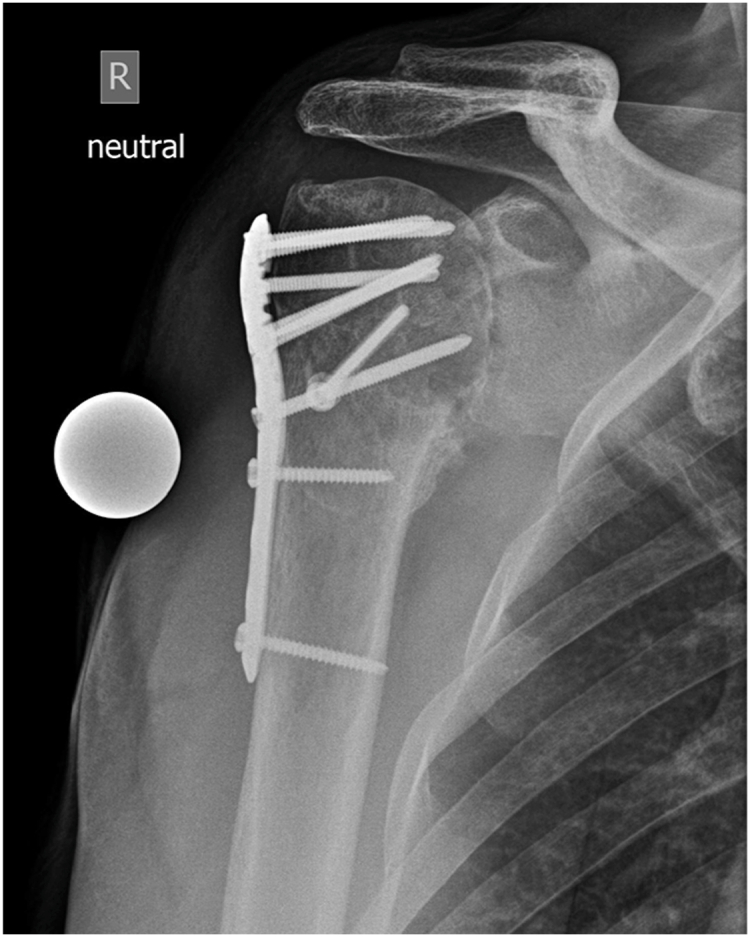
Anteroposterior radiograph of the right shoulder before revision surgery showing glenohumeral osteoarthritis and humeral osteonecrosis.
